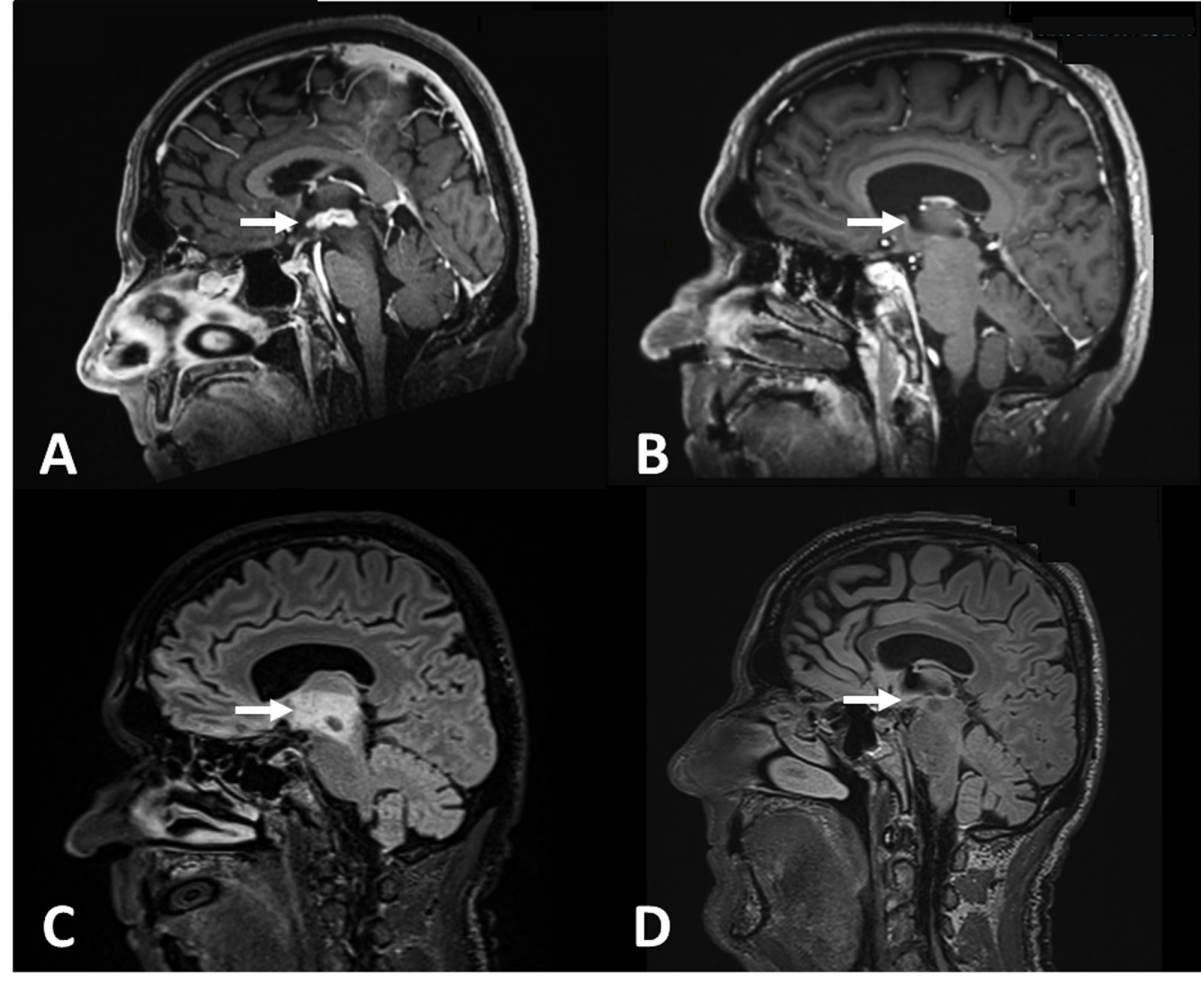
Sagittal MPRAGE MRI post administration of gadolinium contrast agent and T2 FLAIR sequences before and after the patient completed R-CHOP with dexamethasone treatment. A: Sagittal magnetization-prepared rapid acquisition with gradient echo (MPRAGE) MRI shows presence of a large, contrast-enhancing third ventricular lesion. B. Two-week follow up MRI after completion of R-CHOP and steroid treatment, there is a significant reduction in lesion size. C/D: T2 fluid-attenuated inversion recovery (FLAIR) sequences show a similar degree of reduction in the volume of FLAIR hyperintensity.
